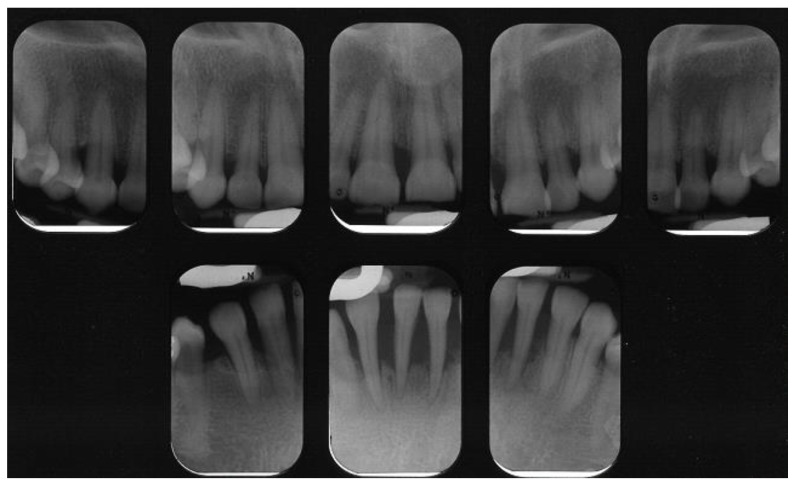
Peri-apical radiographs of patient with Type 2 Diabetes Mellitus. Severe loss of supporting bone is evident. Courtesy of Dr. Brittany Lane.
